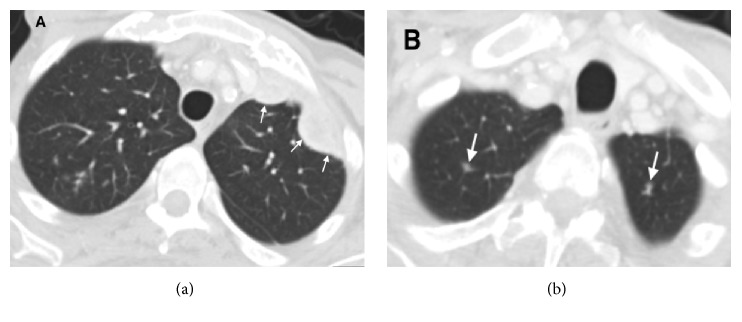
Axial contrast-enhanced corresponding CT scan images after 12 weeks of nivolumab treatment showing a partial response with reduction in size of multiple bilateral lung metastases (white arrows).
